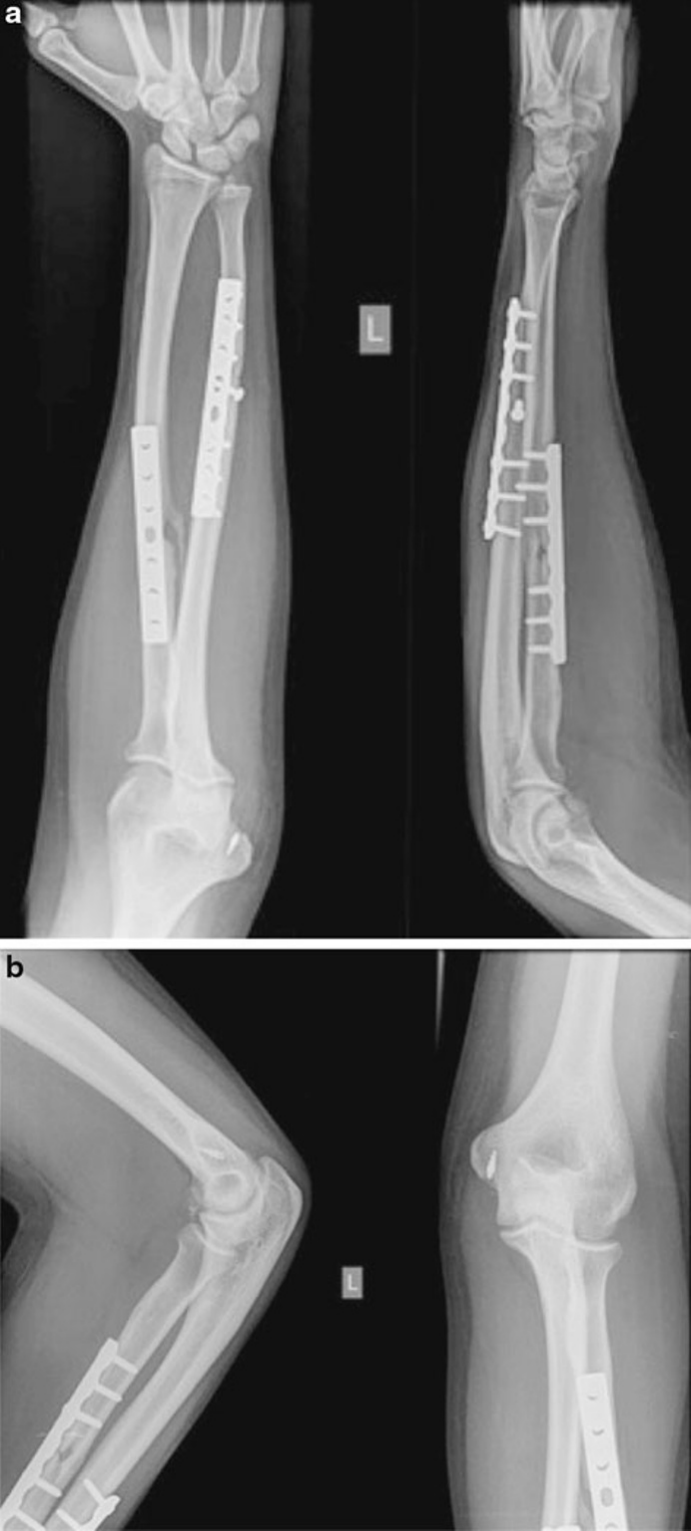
a, b Radiographs of the left elbow and forearm 5 months after the surgery
